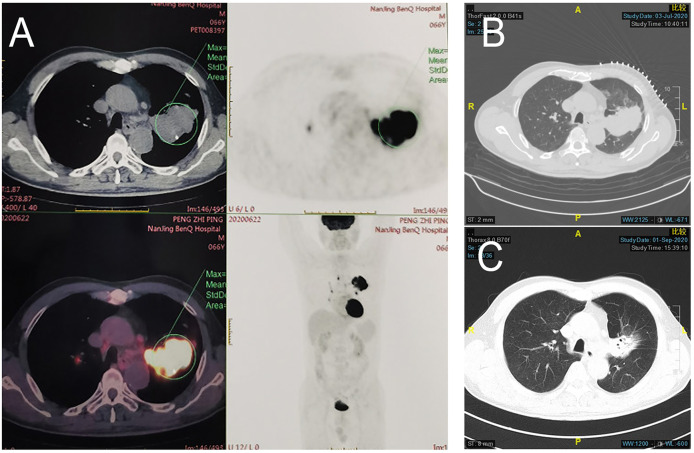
Radiologic examination of Patient 1. (A) Baseline PET/CT showed a mass near the pulmonary hilum of the left upper lung lobe that was approximately 6.7 × 4.2 cm in size with an SUV_max_ of 17.7. Multiple enlarged lymph nodes can be observed at the left lung hilum and mediastinal stations 4 and 5, with an SUV_max_ of 11.1. CT scans of the chest before (B) and after (C) treatment showed shrinking of left upper lung lesions, with radiologic evaluation showing PR (RECIST 1.1).
